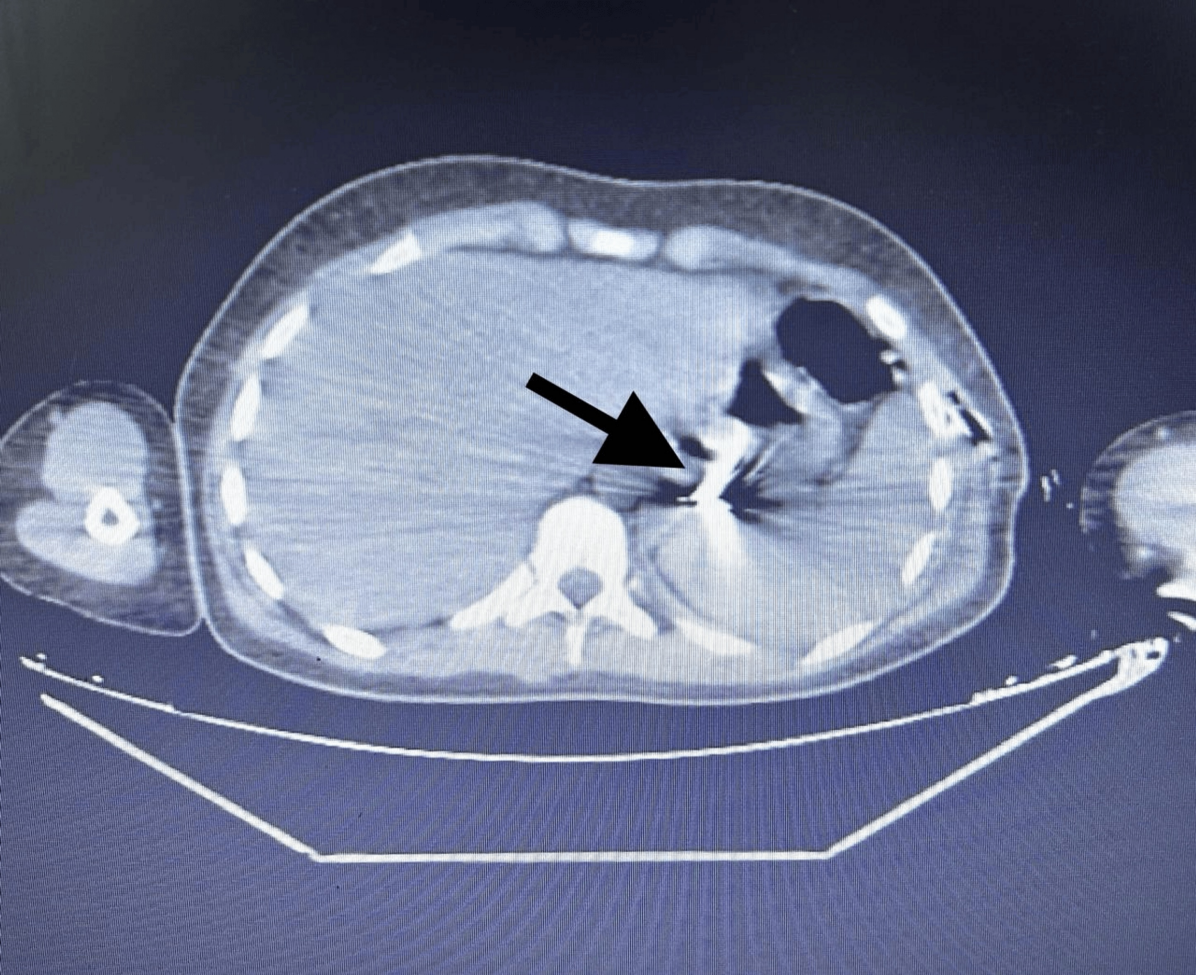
Abdominopelvic CT scan showing a FB in the gastric lumen
